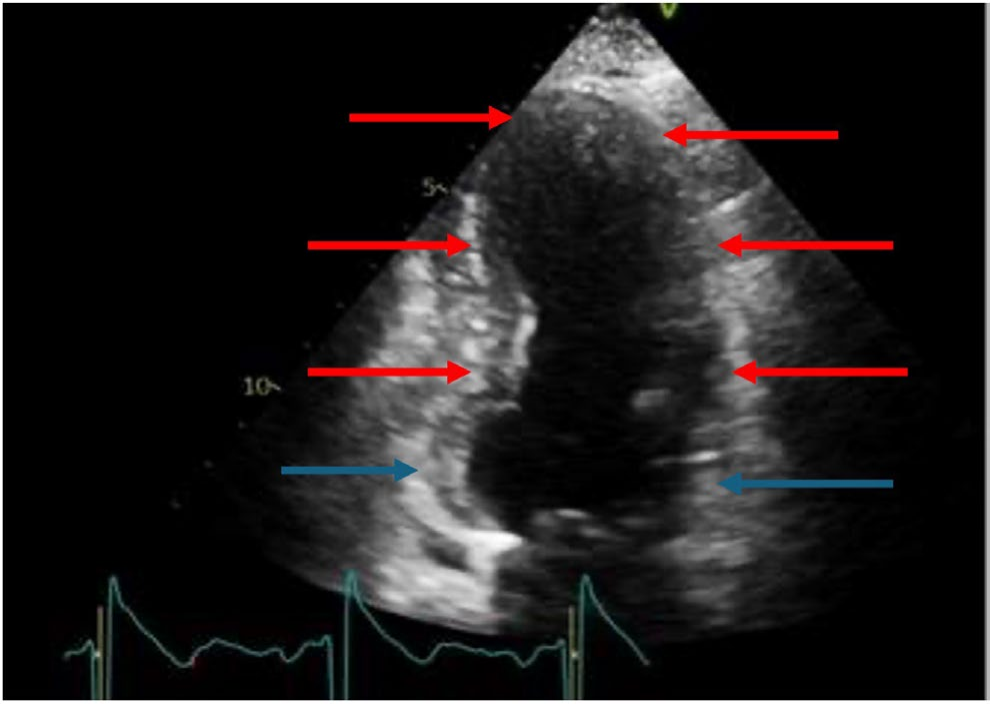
Apical two‐chamber echocardiographic view of the heart demonstrating preservation of the basal segments (blue arrows) while the rest of the myocardium is severely hypokinetic (red arrows). This is not in keeping with a single coronary artery territory and supports the diagnosis of Takotsubo cardiomyopathy, according to the Mayo Clinic diagnostic criteria.
